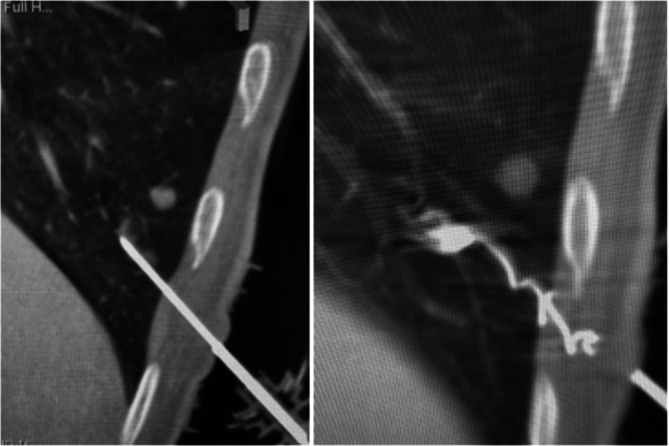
Final CT scan image showing the proximal extremity microcoil accurately positioned within the lung parenchyma at the level of the suspicious nodule and the distal extremity in the extra‐pleural space. The coil's placement is confirmed post‐intervention, with 31.0 mm from the pleura, ensuring precise localization for subsequent surgical resection.
